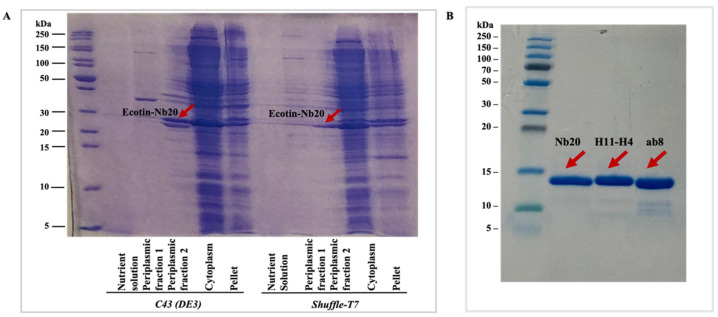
(A) Comparison of the fractions after periplasmic isolation (periplasmic isolation step one and step two), cytoplasm ultrasonication, and assessment of the nutrient solution by SDS-PAGE in the case of the expression of the Nb20 nanobody in *C43(DE3)* and *Shuffle-T7* bacterial strains using the Tac-promoter-based construct. Each sample contains 2.5 μL of sample mixed with 2x SDS loading dye. (B) Comparison of the purified nanobodies by SDS-PAGE in the case of the expression in *Shuffle-T7* bacterial strain using the Tac-promoter-based construct. Each sample contains 2.5 μL of sample mixed with 2x SDS loading dye.
